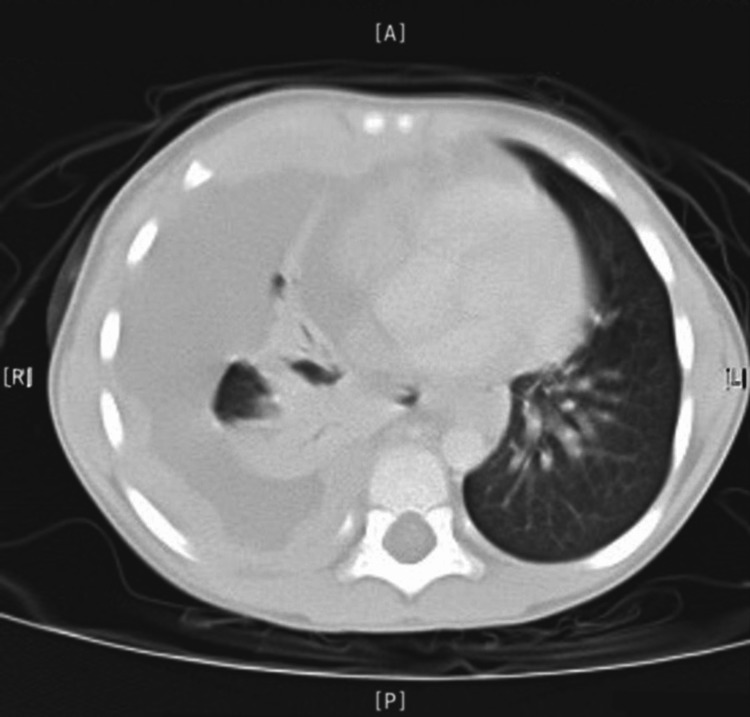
Computed tomography (CT) scan, axial view, lung window shows a marked right-sided pleural effusion, collapsed lung, thick pleural service, shift of mediastinum to the left side.
